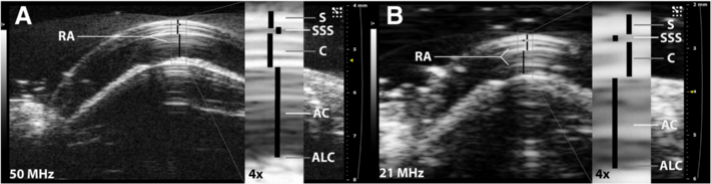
Ultrasonographic images of the eye of a ball python (
